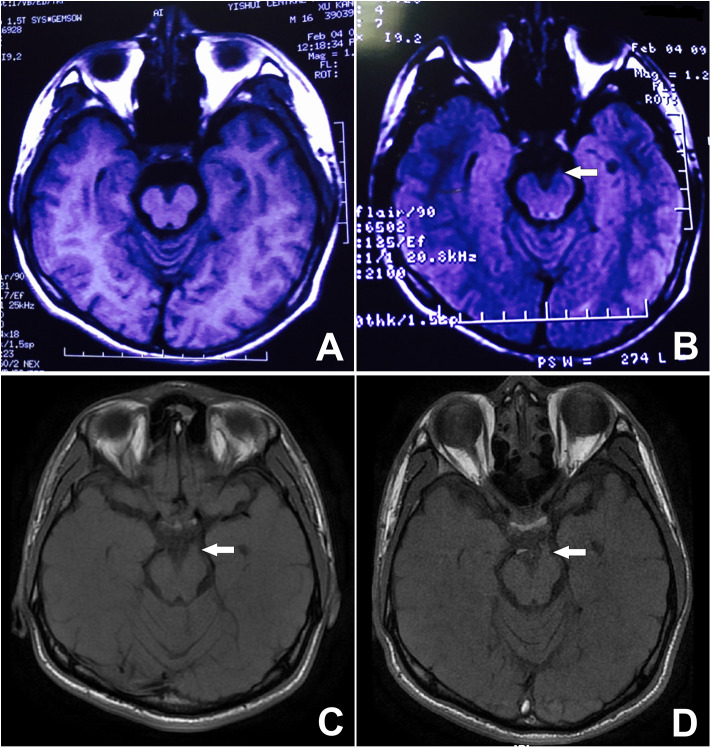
Unenhanced MRI from 9 years prior showing minor thickening of the distal cisternal portion of the left-sided third cranial nerve on T2 FLAIR. See arrow (B). The signal on T1 is obvious, during both the attack and remission. See arrow (C,D) although it is not visible on the T1 image from 9 years ago (A).
